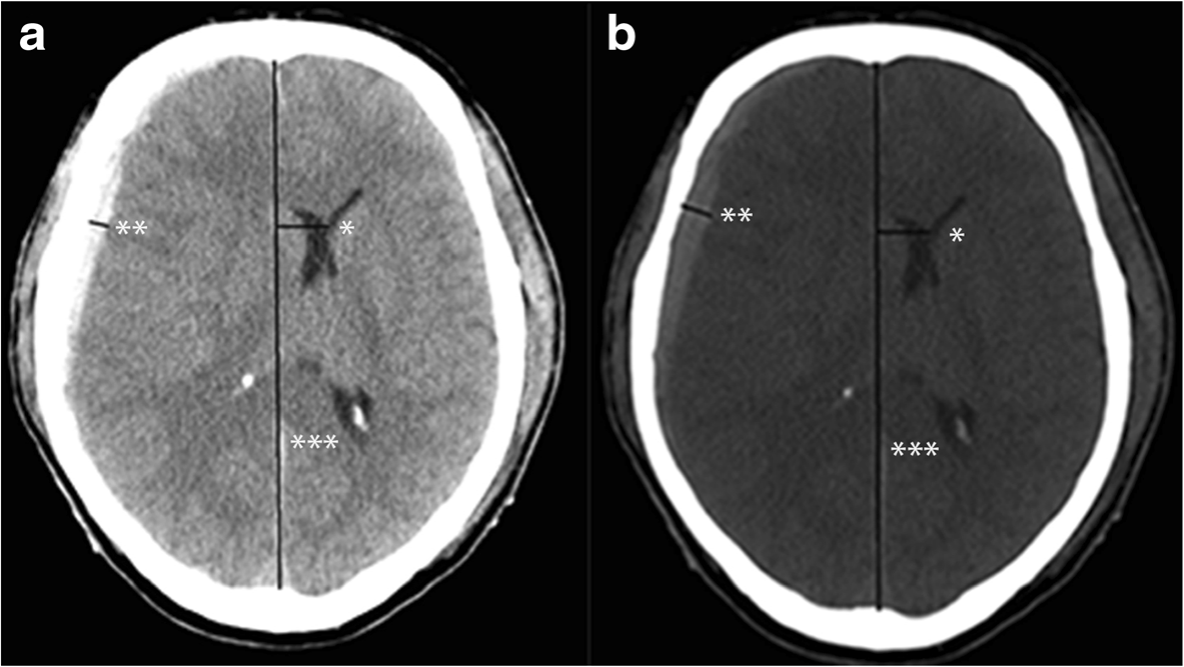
Depicting a CT scan of a patient who suffered from a right sided acute subdural hematoma. The standard windows W/L is shown in (a), and also the method to measure the thickness of the hematoma () and the midlineshift (midline *, shift *). The thickness of the hematoma was 5 mm and the MLS 15 mm. After adapting the windows W/L was to the suggested level (b) the thickness of the hematoma was 10 mm and the MLS 15 mm
